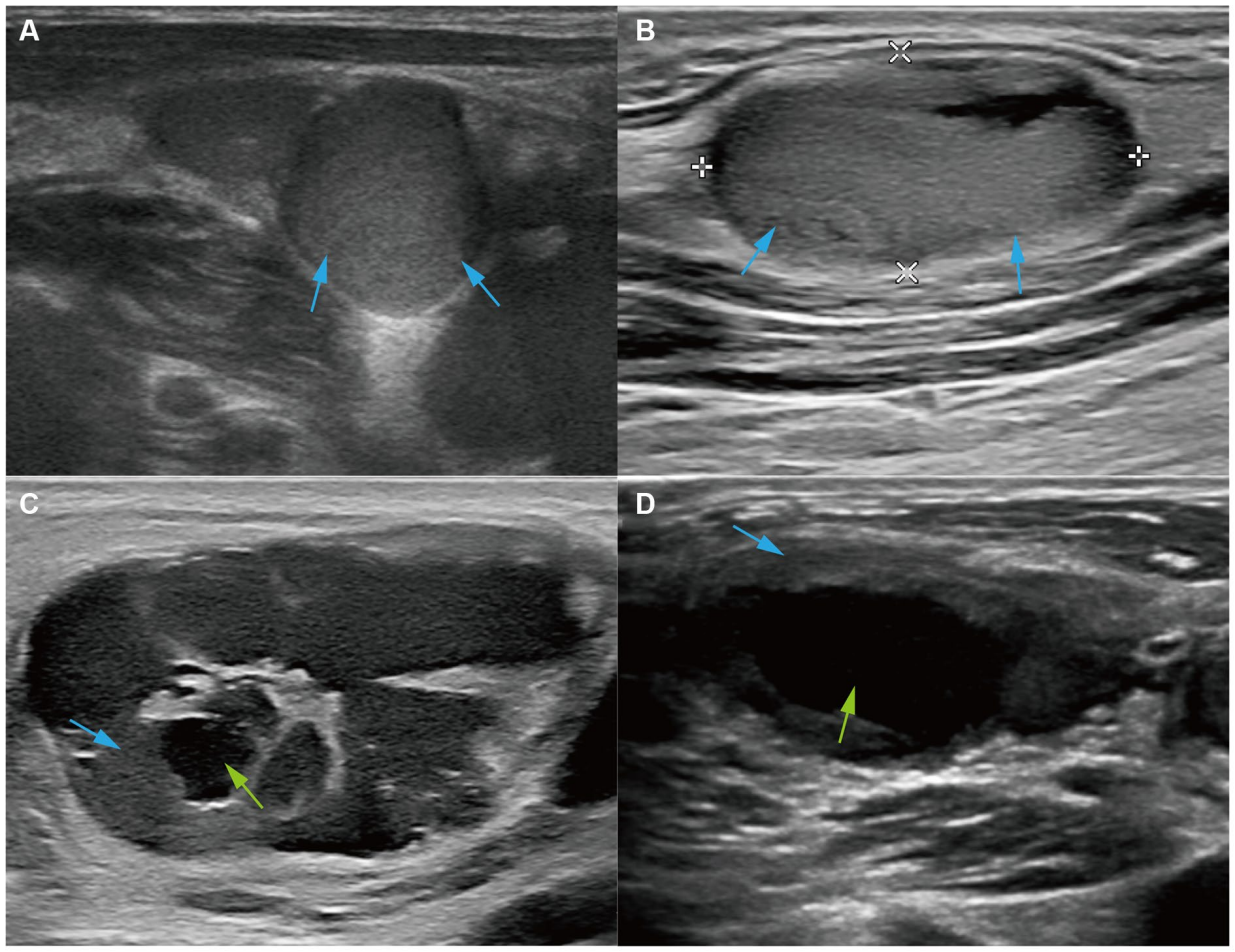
Ultrasound features of echogenicity. Ultrasound images of (A) the right cervical LN from a 37-year-old patient and (B) the left cervical LN from a 29-year-old patient showed that LNs were hyperechoic (red arrows). Ultrasound images of (C) the left cervical LN from a 29-year-old patient and (D) the left cervical LN from a 33-year-old patient showed hyperechoic LNs (red arrows) and large liquefaction (green arrows).
